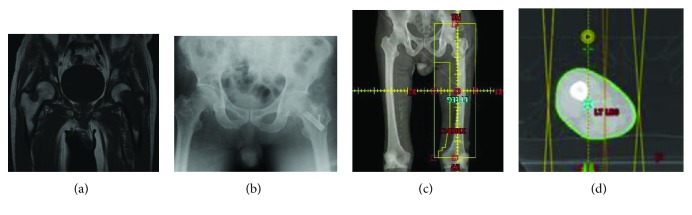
(a) Coronal T1 MR image showing extensive disease in the left femoral head, neck, and proximal metaphysis without an actual fracture of the bone. A contralateral lesion is seen in the superolateral aspect of the right femoral neck with several deposits along the femoral shaft. (b) AP pelvic X-ray showing prophylactic nailing with a CFR-PEEK cephalomedullary nail. (c, d) CT planning images for radiotherapy with the absence of metal artefacts.
